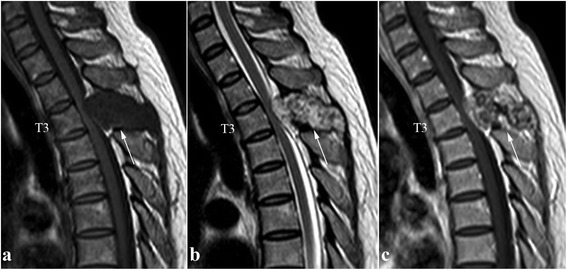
Preoperative sagittal T1-weighted (a) and T2-weighted (b) MR images showing a well-circumscribed extradural mass, which was homogeneous hypointense on T1-weighted images and mixed iso- to hyperintense on T2-weighted images. Administration of Gd-DTPA resulted in heterogeneous enhancement on T1-weighted images (c) (*white arrows*)
